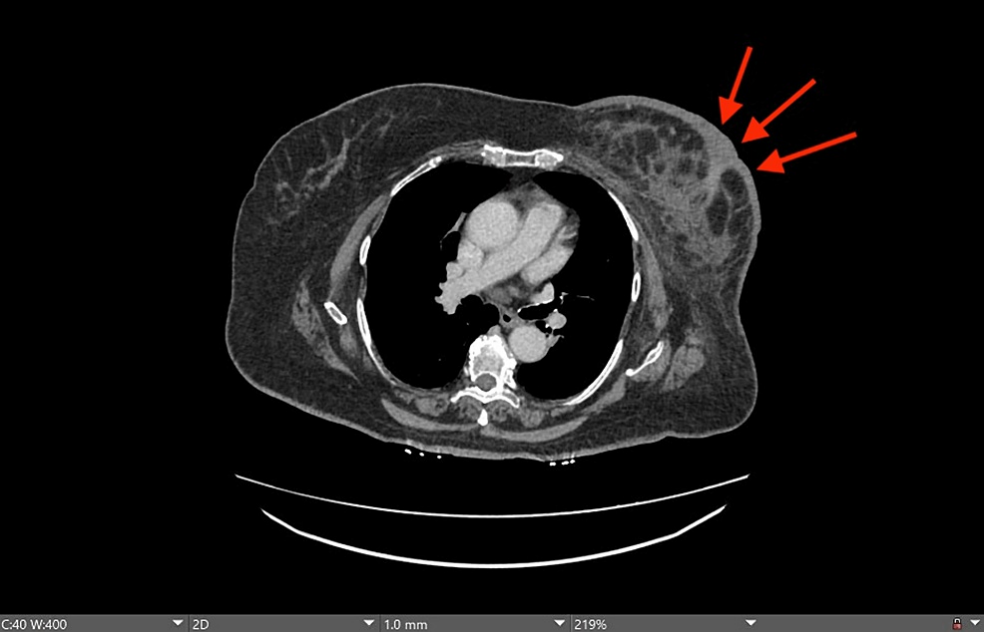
CT scan at the level of T4. Axial contrast-enhanced image demonstrating enhancement and skin thickening of the left breast. The edema and skin thickening are related to prior treatment changes based on the radiologic impression. Red arrows point to the site of the lesion.
